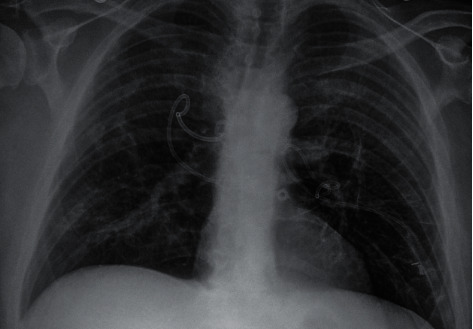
Anteroposterior chest radiograph taken 1 week after chest tube placement.
